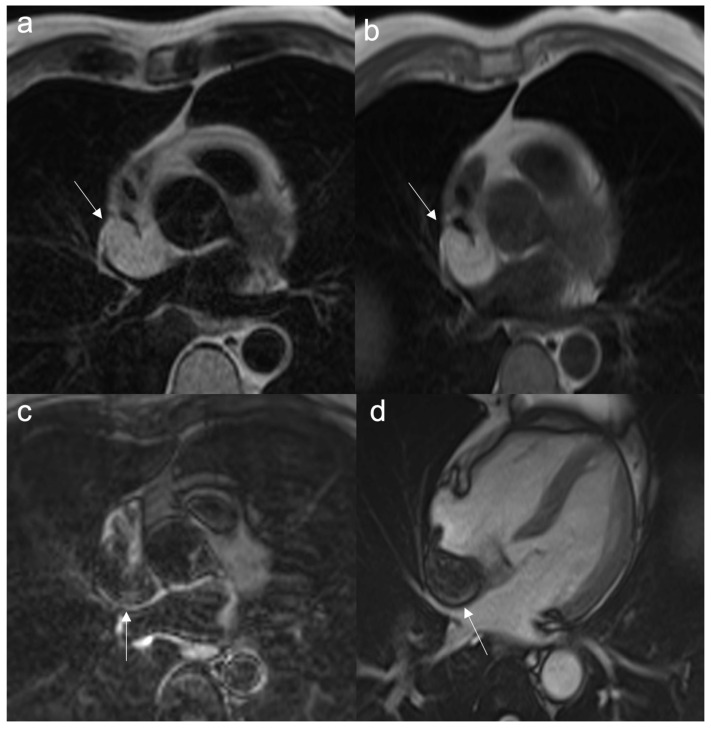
Lipomatous hypertrophy of the interatrial septum (arrow): a 70-year-old woman with acute chest pain arrived at the Emergency Department; a CT scan shows a lung mass suspected of cancer and a hypodense lobulated mass, with densitometric values similar to lipid, extending from the atrial septum upward to the outlet of the SVC in the right atrium. The mass is hyperintense on both MRC T1w and T2w sequences (a,b), has signal loss in T2w Fat Sat due to its lipid content (c), and appears in a typical “dumbbell’’ shape in 4CH SSFP sequences (d).
